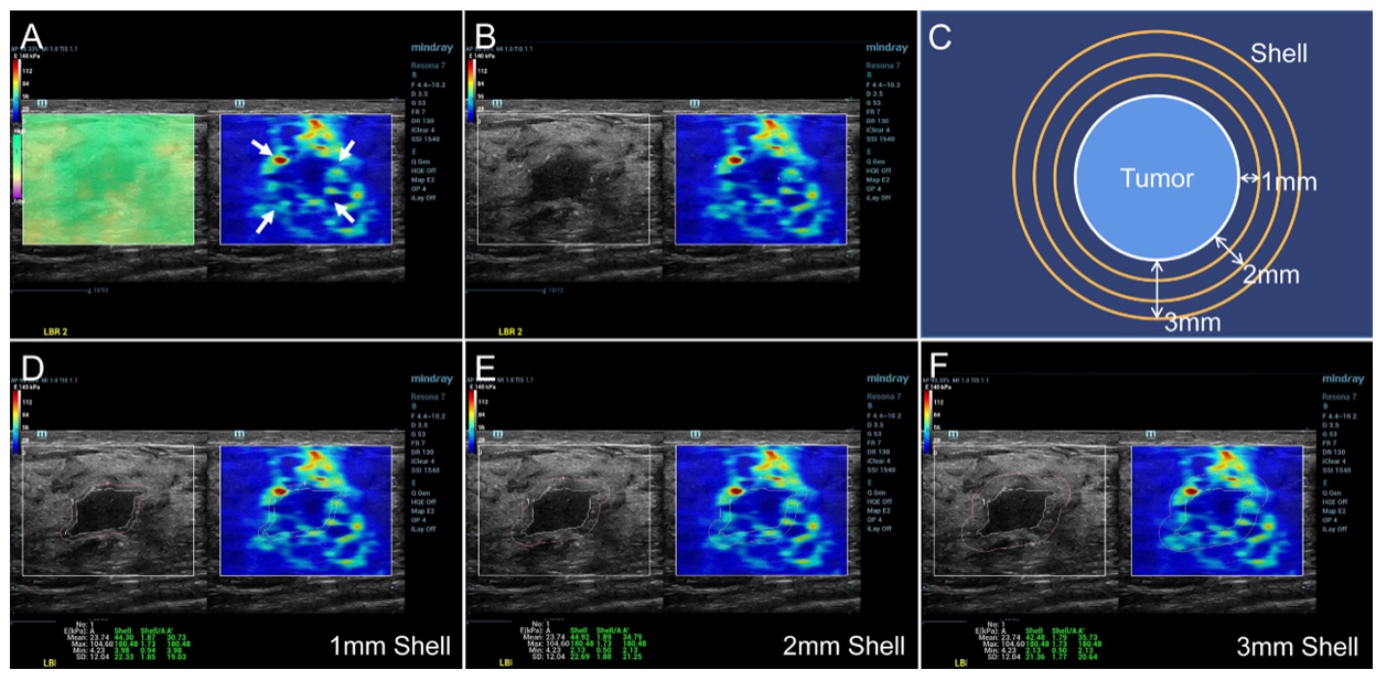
SWE dual dynamic mode of invasive ductal carcinoma. A satisfied SWE image quality was obtained before quantitative analyses (Figure 2A left, represented as uniform green). The red and orange halo around the tumor suggested the stiff rim sign (Figure 2A right, marked as arrows). The maximum diameter of lesion was then measured in the SWE dual dynamic mode (Figure 2B). A sketch map of the stiff rim sign was presented in Figure 2C. The ultrasound system would automatically plot the shells around the tumor with a width of 1-3 mm. When the tumor boundary was delineated manually on the gray-scale map, the system calculated the YM values of the lesion, 1-3 mm shell, and the area contained the lesion and the shell (Figure 2D-F). SWE: shear wave elastography, YM: Young's modulus.
